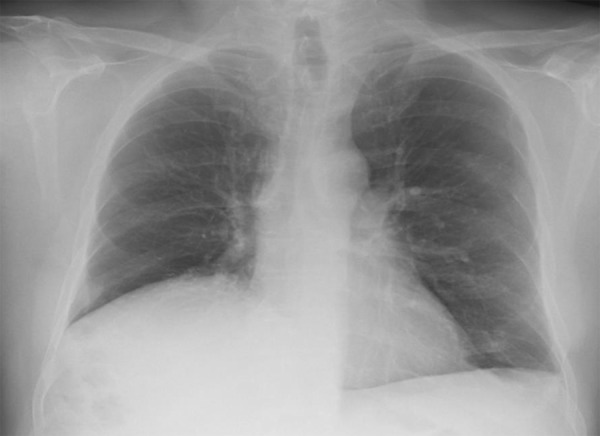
Chest radiograph demonstrating raised hemidiaphragm.
